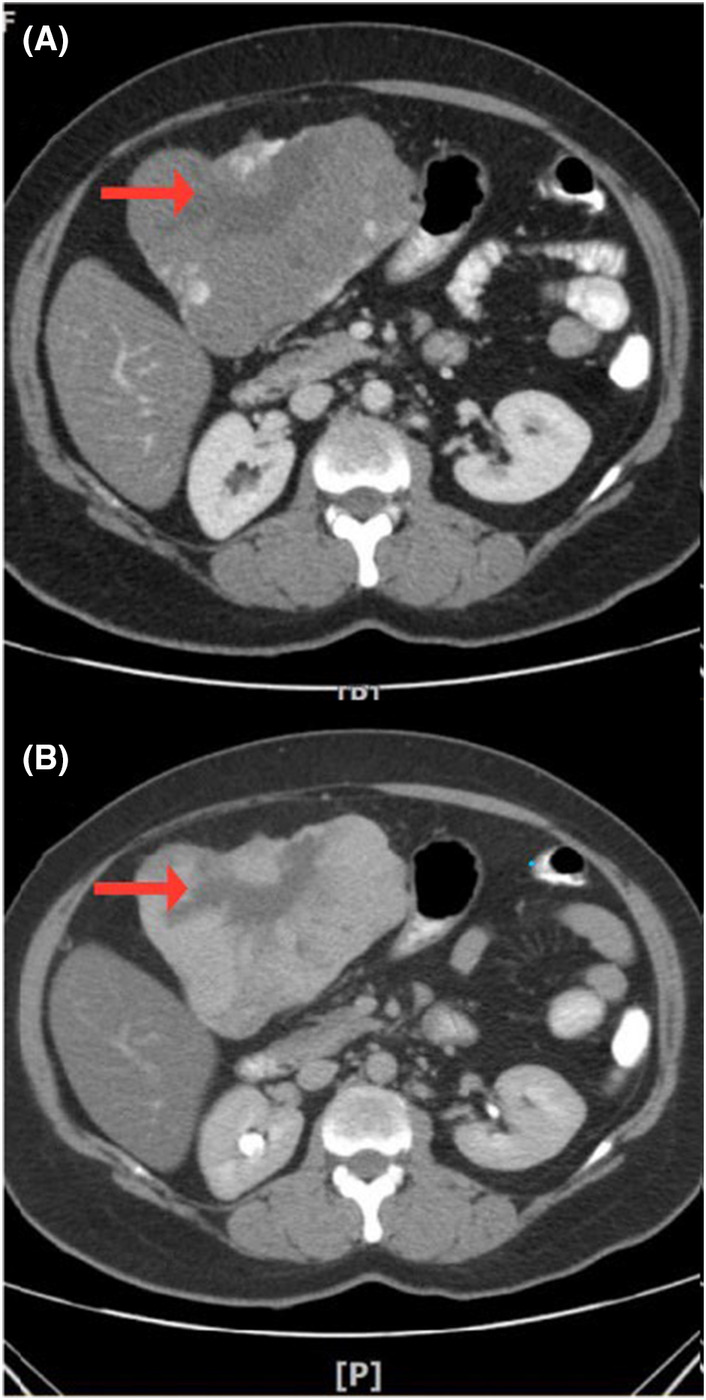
The red arrow indicates the probable central hemorrhage (A) portal phase of CT scan: Intense nodular marginal enhancement is noticed. (B) Latent phase of CT scan: a fill‐in pattern of enhancement is noticed, and most of it, except the inner areas, is hyper‐dense and have the same density as blood vessels, which recommends giant liver hemangioma.
